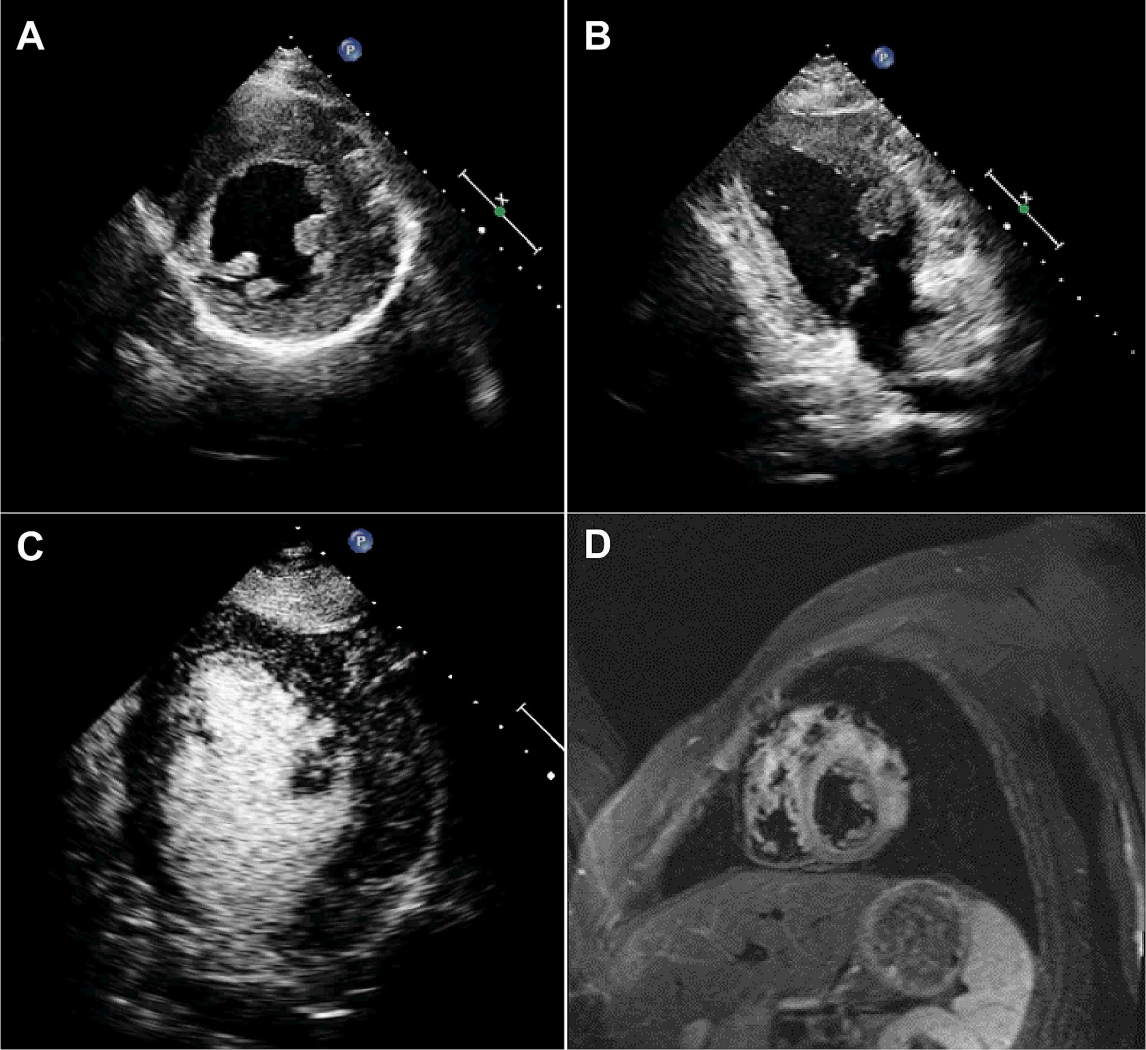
(A, B) Abnormal masses can be seen in the epicardial layer of the lateral wall of the left ventricle, with strong echoes and dark areas separated by radial ribbons. The boundary with normal myocardial echo is still clear, and the left ventricular normal myocardial hypertrophy is mild. (C) The left ventricular wall is significantly thickened, and the abnormal echogenicity of the left ventricular lateral wall appears to be accompanied by contrast agent echo. (D) MRI revealed uneven thickening of the myocardium, local nodular formation, and diffuse edema, ultimately confirmed by surgery as myocardial cavernous hemangioma
